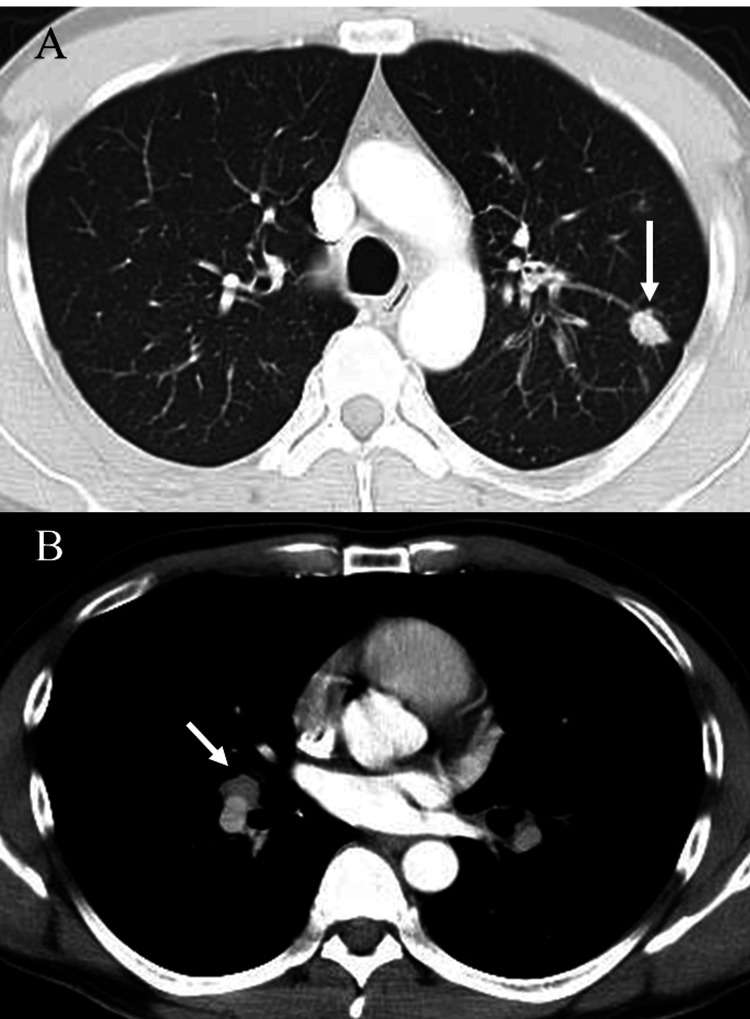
The CT scans obtained at the previous hospital Initial chest CT showed a solitary nodule in the left upper lung lobe (A, arrow) and bilateral hilar and mediastinal lymphadenopathy (B, arrow).
